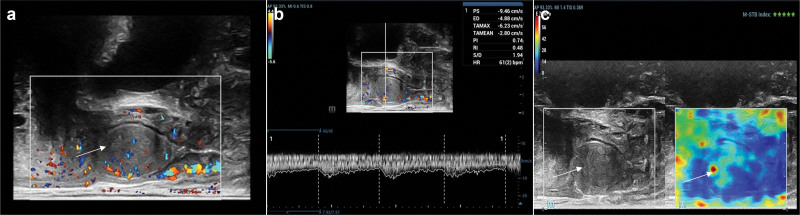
TRBU with elastography. The white arrow indicates the vaginal mass. (A and B) Abundant blood flow signals were detected within the mass, with a vascular RI of 0.48, regular vascular structure, and relatively uniform distribution; (C) elastography showed that the central region of the mass exhibited a local red area in terms of tissue hardness distribution, while the peripheral area showed a gradual decrease in hardness, transitioning from yellow to green. RI = resistance index, TRBU = transrectal biplane ultrasonography.
